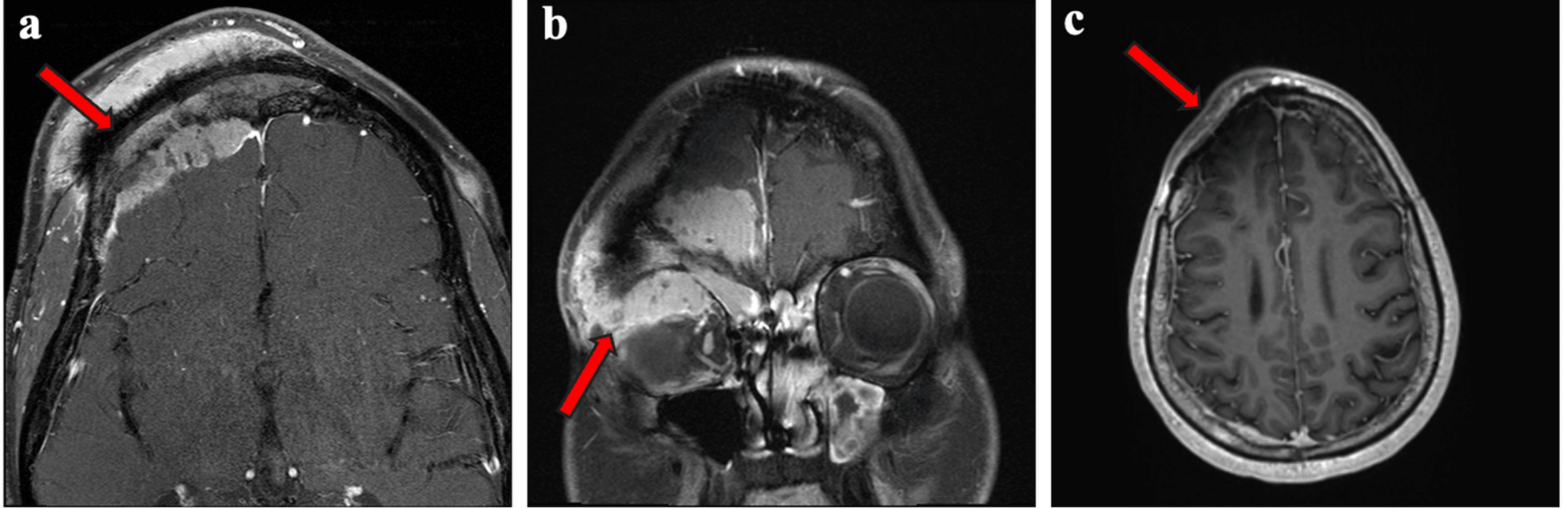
Post-contrast T1-weighted brain MRI (October 2024). The red arrows indicate enhancing lesions: (a) an avidly enhancing 3.4 × 7.0 × 4.7 cm transcalvarial right frontal mass extending from the scalp through the frontal bone/frontal sinus into the right frontal epidural space; (b) a 1.4-cm-thick enhancing mass in the superior right orbit centered at the prior right frontal/supraorbital cranioplasty; and (c) an enhancing right parietal calvarial lesion (~3.6 cm) with adjacent dural thickening/enhancement (up to 0.5 cm).
